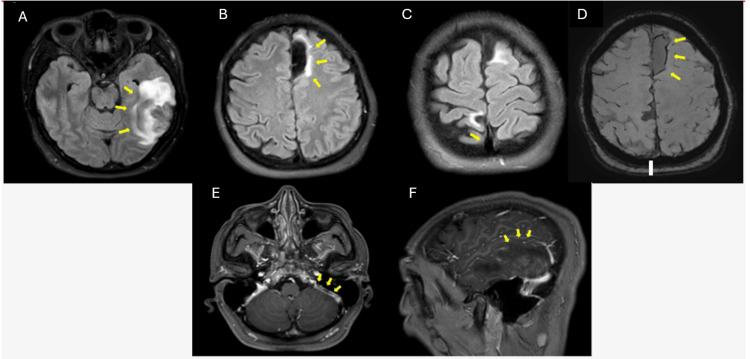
Brain MRI findings (A) Axial FLAIR sequence demonstrating vasogenic edema predominantly in the left temporal lobe, with associated hemorrhagic components (yellow arrows), consistent with hemorrhagic venous infarction. (B-C) Areas of encephalomalacia and gliosis involving the left frontal lobe (B) and the right postcentral gyrus (C) (yellow arrows), suggestive of prior ischemic or hemorrhagic events. (D) Axial SWI showing superficial siderosis over the left frontal convexity, indicative of a previous hemorrhagic insult. (E-F) Post-contrast 3D MRI (axial and sagittal views): (E) filling defect within the left sigmoid sinus (arrow), consistent with subacute venous thrombosis, and (F) absence of flow in the vein of Labbé and its cortical tributaries (yellow arrows), confirming cortical venous involvement. Overall, these findings are consistent with extensive cerebral venous thrombosis involving the left sigmoid sinus and the vein of Labbé, associated with a hemorrhagic venous infarction in the left temporal lobe. MRI: magnetic resonance imaging; FLAIR: fluid-attenuated inversion recovery; SWI: susceptibility-weighted imaging
